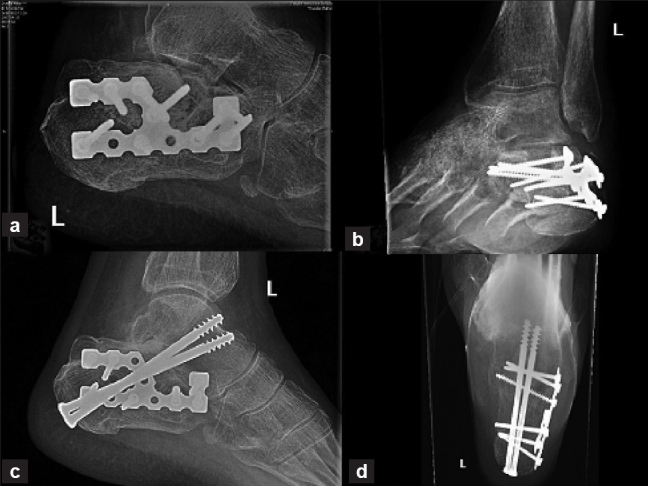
X-rays of ankle joint (lateral and oblique views) showing imperfect reconstruction of cancaneal shape, decreased bohler's angle (a) and non reduced posterior facet (b), which lead to post traumatic arthritis pain. X-ray of ankle joint (lateral and anteroposterior view) showing subsequent arthroscopically assisted reconstructive isolated subtalar distraction tricortical arthrodesis (c, d).
